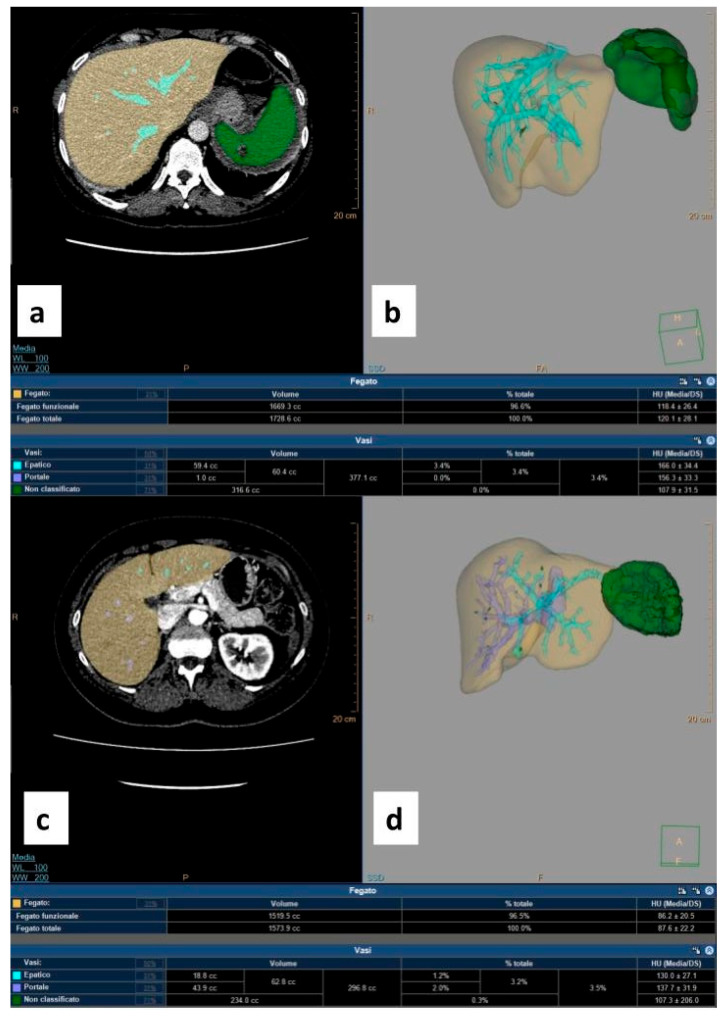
Three-dimensional reconstruction CT-based images obtained using IntelliSpace software v. 12.1. (a) Pre-procedural contrast-enhanced axial CT scan acquired during the venous phase shows the hepatic parenchyma in yellow and the healthy splenic parenchyma in green; lacerated–contused areas are not colored. (b) Pre-procedural volumetric rendering of the splenic parenchyma in green with the corresponding result in the table (healthy splenic volume: 316.6 cc). (c) Post-splenic artery embolization (SAE) contrast-enhanced axial CT scan acquired during the venous phase shows the hepatic parenchyma in yellow and the residual healthy splenic parenchyma in green. (d) Post-SAE volumetric rendering of the splenic parenchyma in green with the corresponding result in the table (residual splenic volume: 234 cc).
